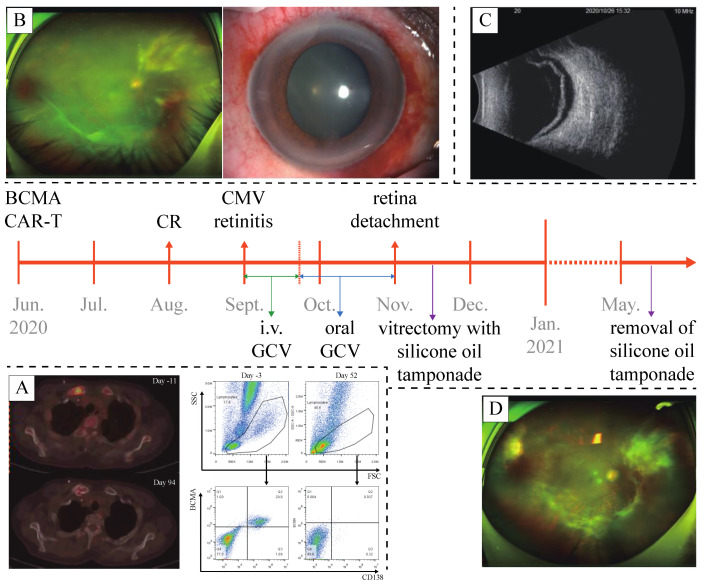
A timeline of the case. (A) Post-CAR-T flow cytometry of bone marrow sample and PET-CT confirmed the complete remission of multiple myeloma. (B) Anterior segment examination and fundoscopy at the diagnosis of CMV retinitis. (C) Ultrasonography of the right eye confirmed retinal detachment. (D) Fundoscopy after the removal of silicone oil tamponade, showing recovery from CMV retinitis and retinal detachment.
